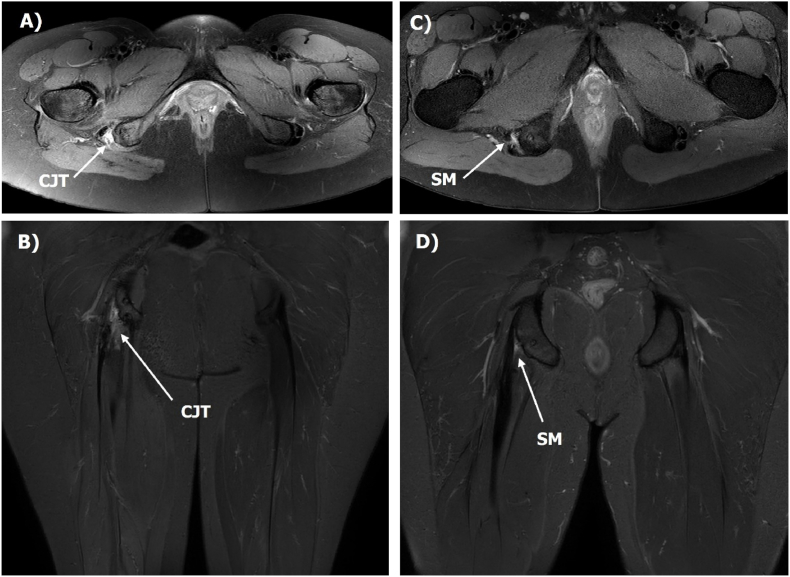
Proximal Hamstring Objective Magnetic Resonance Imaging Score (PHOMRIS) images representing: (A) axial and (B) coronal plane images of the conjoint (CJT) scored at 5, and (C) axial and (D) coronal plane images of the semimembranosus (SM) scored at 2.
